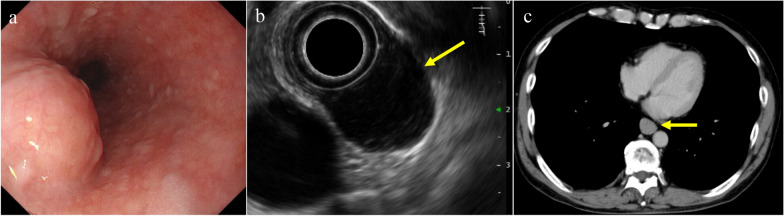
Preoperative findings. a Submucosal tumor of 18 mm × 17 mm was observed on the left wall, 35 cm from the upper incisors in the esophagogastroduodenoscopy. b Circular tumor continuous with the fourth layer was observed in endoscopic ultrasonography (yellow arrow). c 16 mm long nodule was found in the lower esophagus (yellow arrow)
